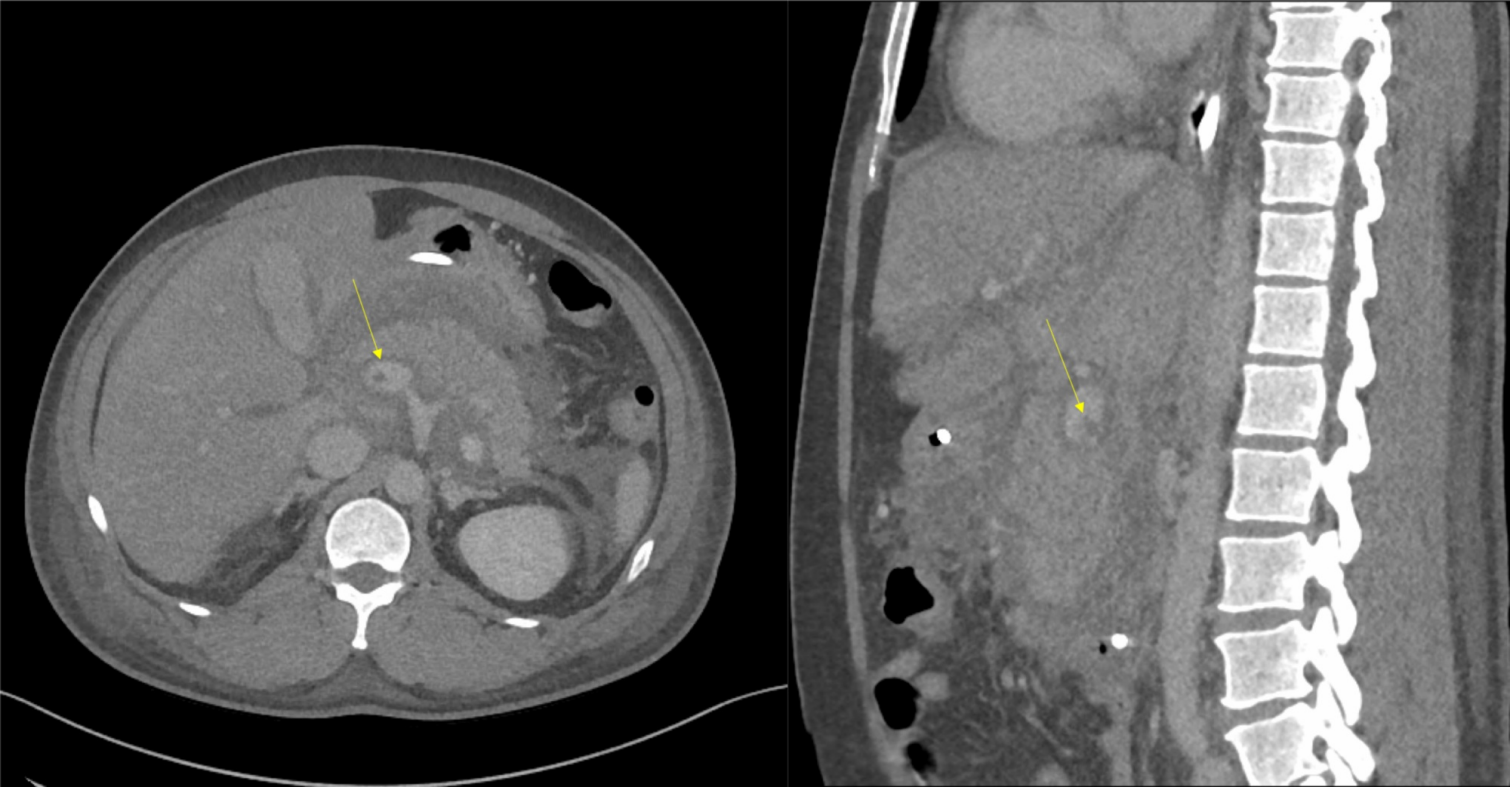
Repeat abdominal CT scan with contrast The image shows partial portal vein thrombosis (yellow arrows) CT: computed tomography
